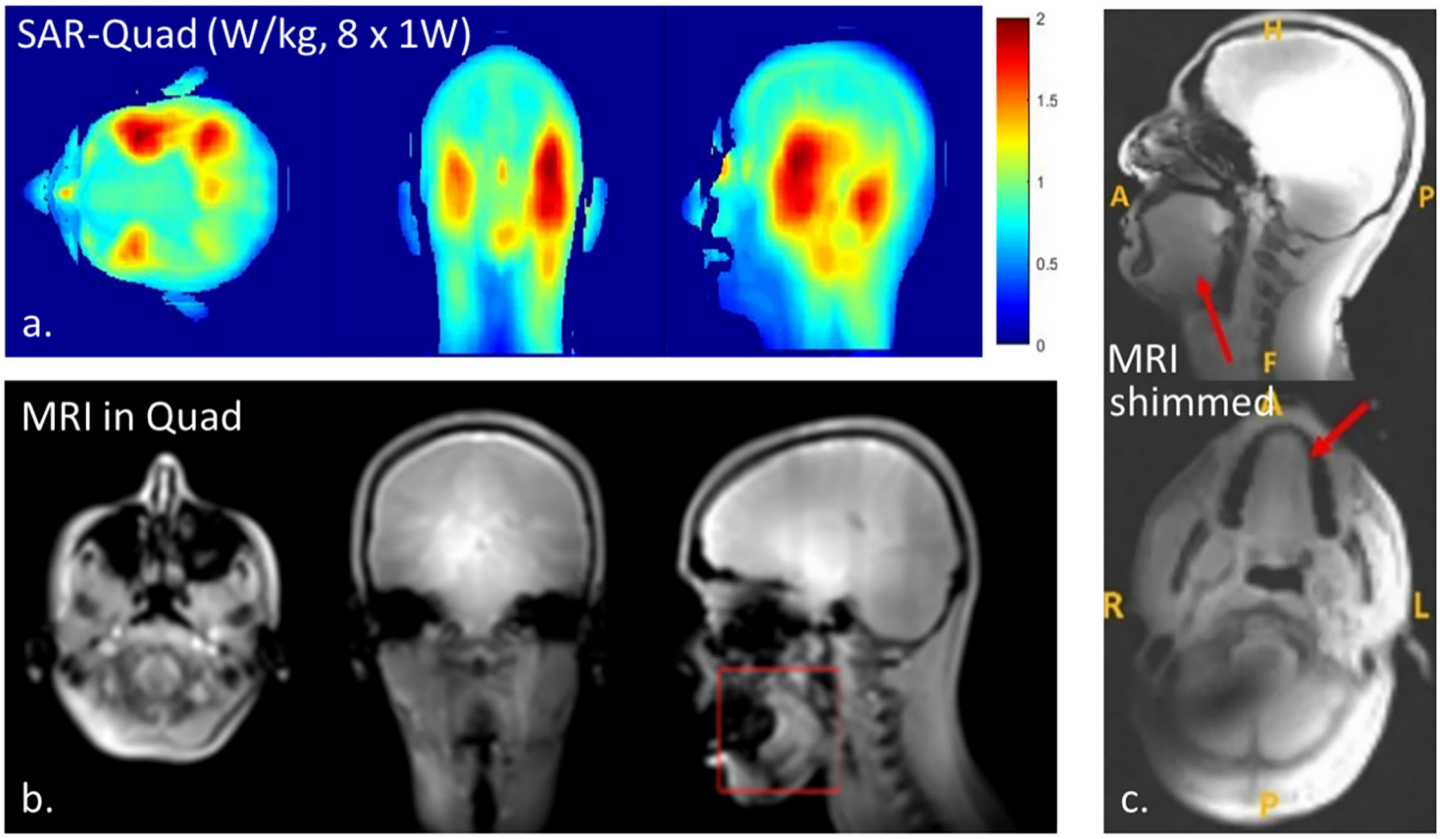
Results with the ^1^H dipole array indicating RF power deposition when driven in quadrature (a), anatomical MRI when driven in quadrature (b), and anatomical MRI when using constructive B${}_{1}^{+}$ interference (RF shimming, c). Note that RF shimming in the tongue will result in B${}_{1}^{+}$ shading in certain areas of the brain (c).
